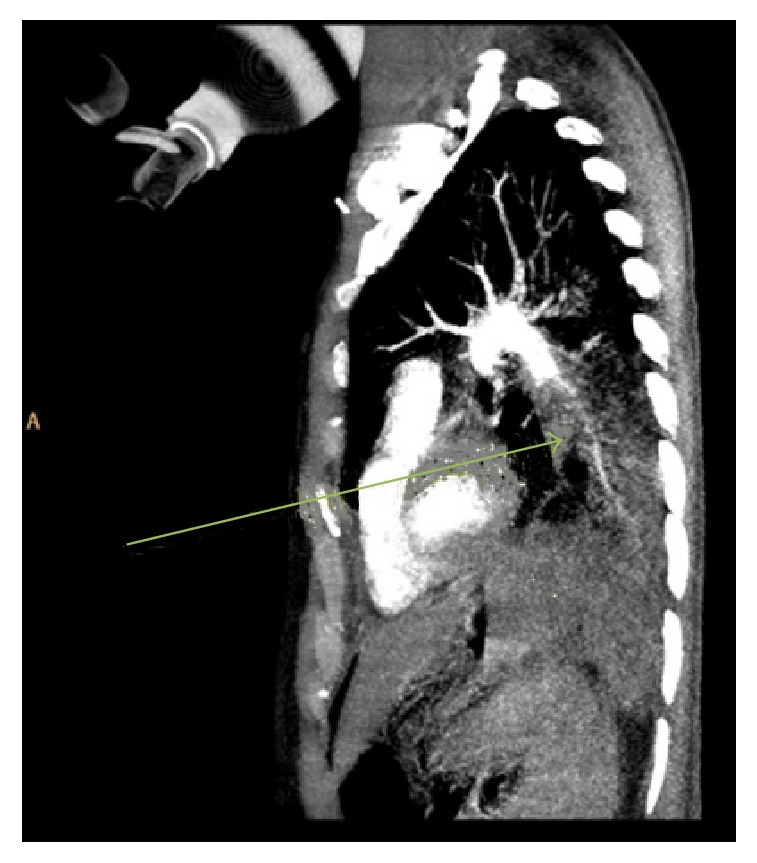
Left parasagittal contrast enhanced computed tomography pulmonary angiography. The arrow points to a large filling defect (pulmonary embolus) in the left pulmonary artery.
